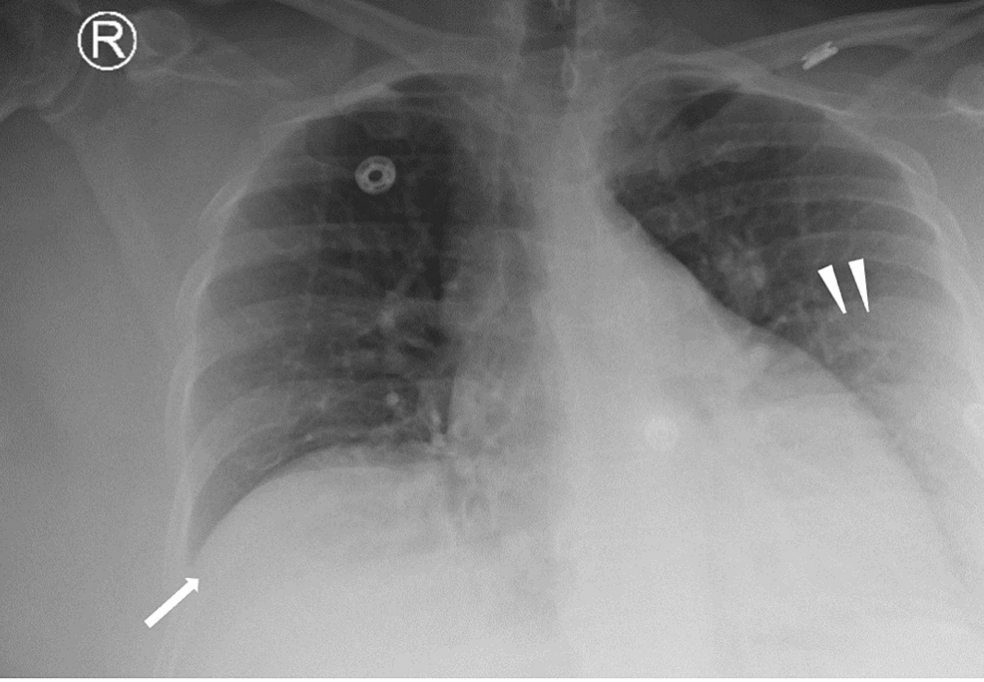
Chest X-ray displaying a mild elevation of the hemidiaphragm (arrows) Haziness along the lateral left mid to lower lung field is artifactual secondary to overlying soft tissue (arrowheads).
